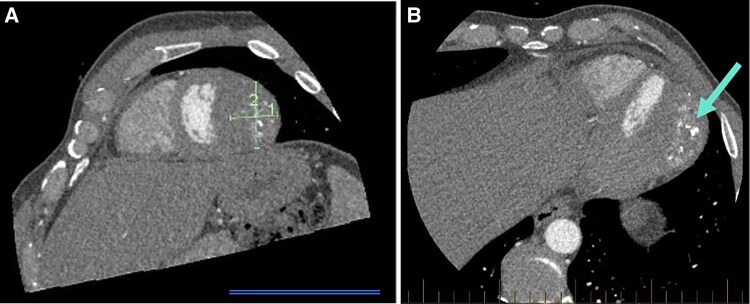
Computed tomography coronary angiogram, axial images displaying a 42 × 30 × 58 mm cardiac mass (*A*). (*B*) The complexity of the lesion with areas of calcification (blue arrow) and demonstrating the difficulty delineating whether arising from pericardium or myocardium.
